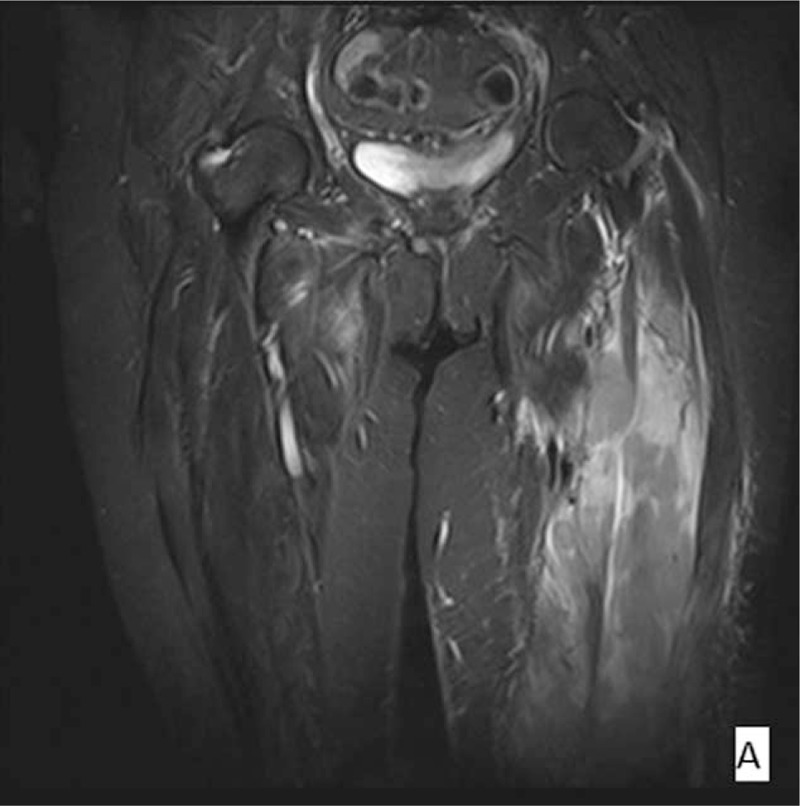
Coronal fat-suppressed T2-weighted image shows an infiltrating soft tissue mass of high signal intensity involving the anterior and lateral compartment of left femur and the internal adductor muscles of the right femur. Subcutaneous stranding is present.^[4]^
